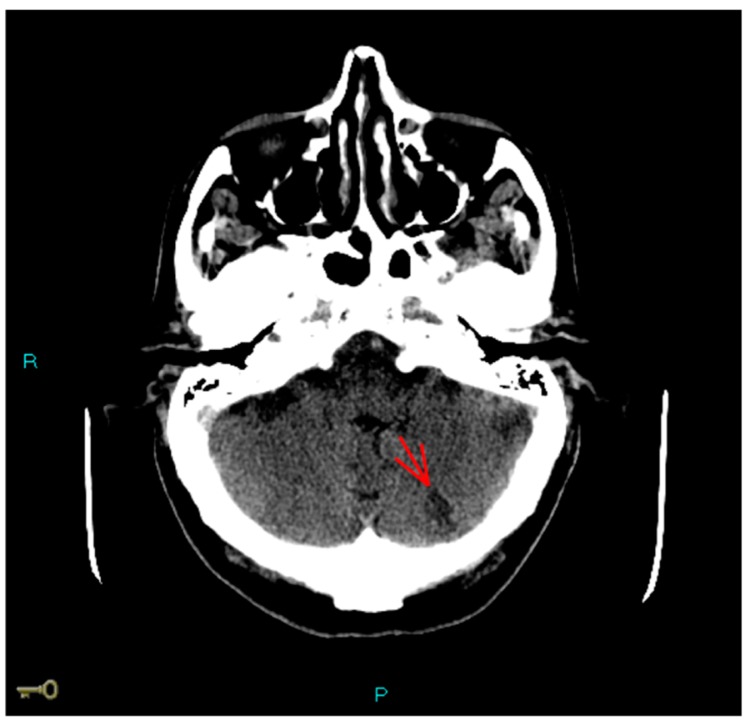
Angio-CT (computational tomography) brain scan. The exam showed an acute ischemic lesion in the left cortico-subcortical temporo-occipital area and in the left cerebellar hemisphere (last one marked with red arrow).
